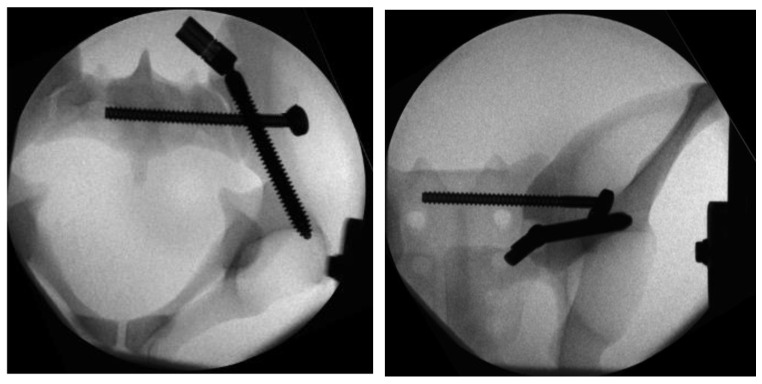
Radiographs in two planes of the conventional triangular fixation with the sacroiliac screw separate from the iliac screw in a more cranial position.
